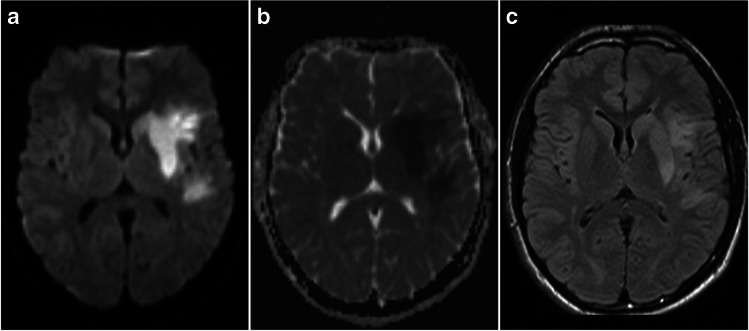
DWI and FLAIR images acquired in the phase of extracellular oedema. Images of the same patient as in Fig. 4, acquired 21 h later. a The image shows an increased signal on DWI with b a corresponding signal drop on ADC as well as c a clearly visible compatible high signal area on FLAIR
